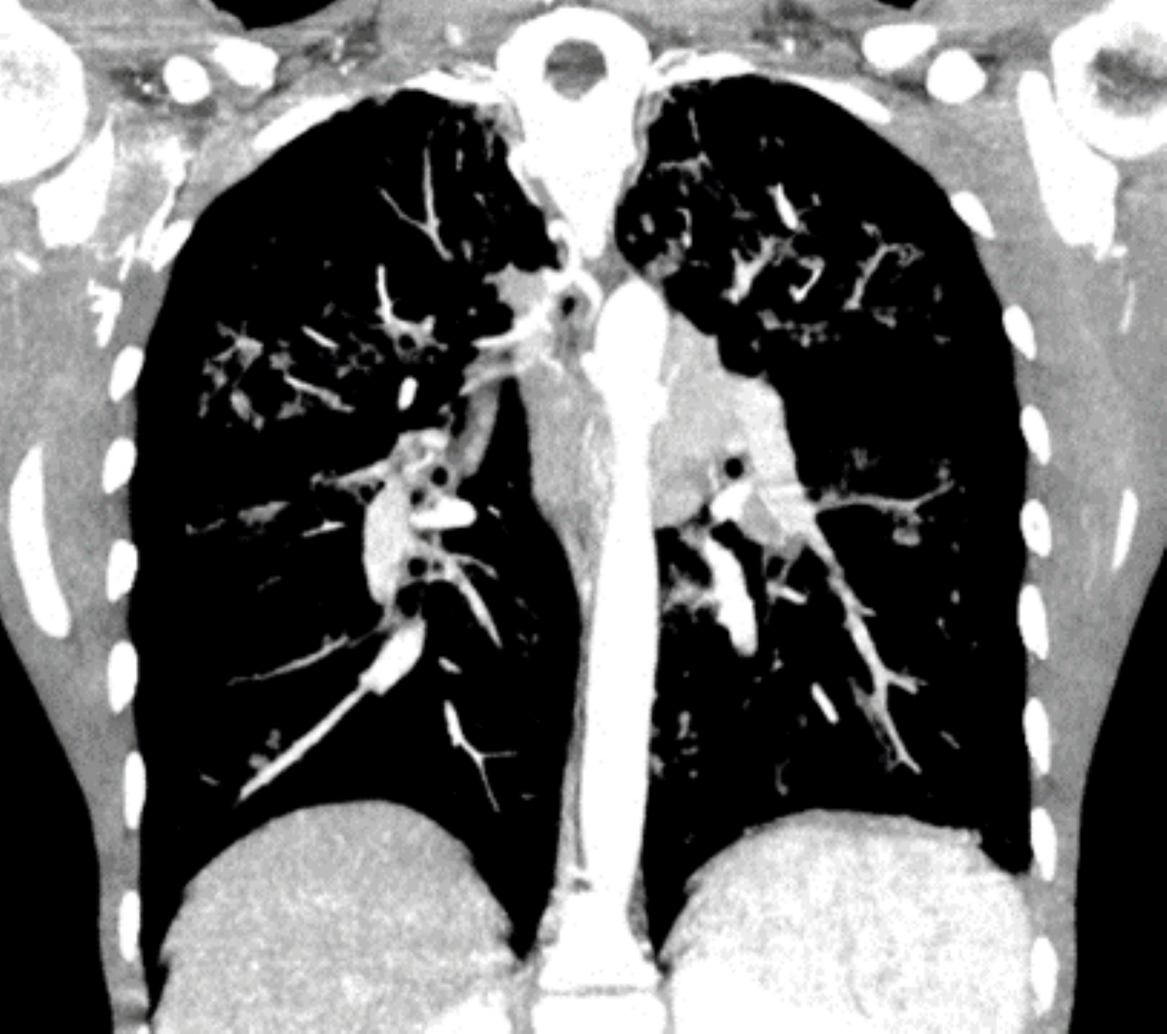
Patient's last CT scan (March 2023) CT scan showed multiple bronchiectasis features dispersed across all pulmonary lobes, ectasia of the bronchial arteries, and multiple hilar and mediastinal adenomegalies, among other findings. CT: Computed tomography
